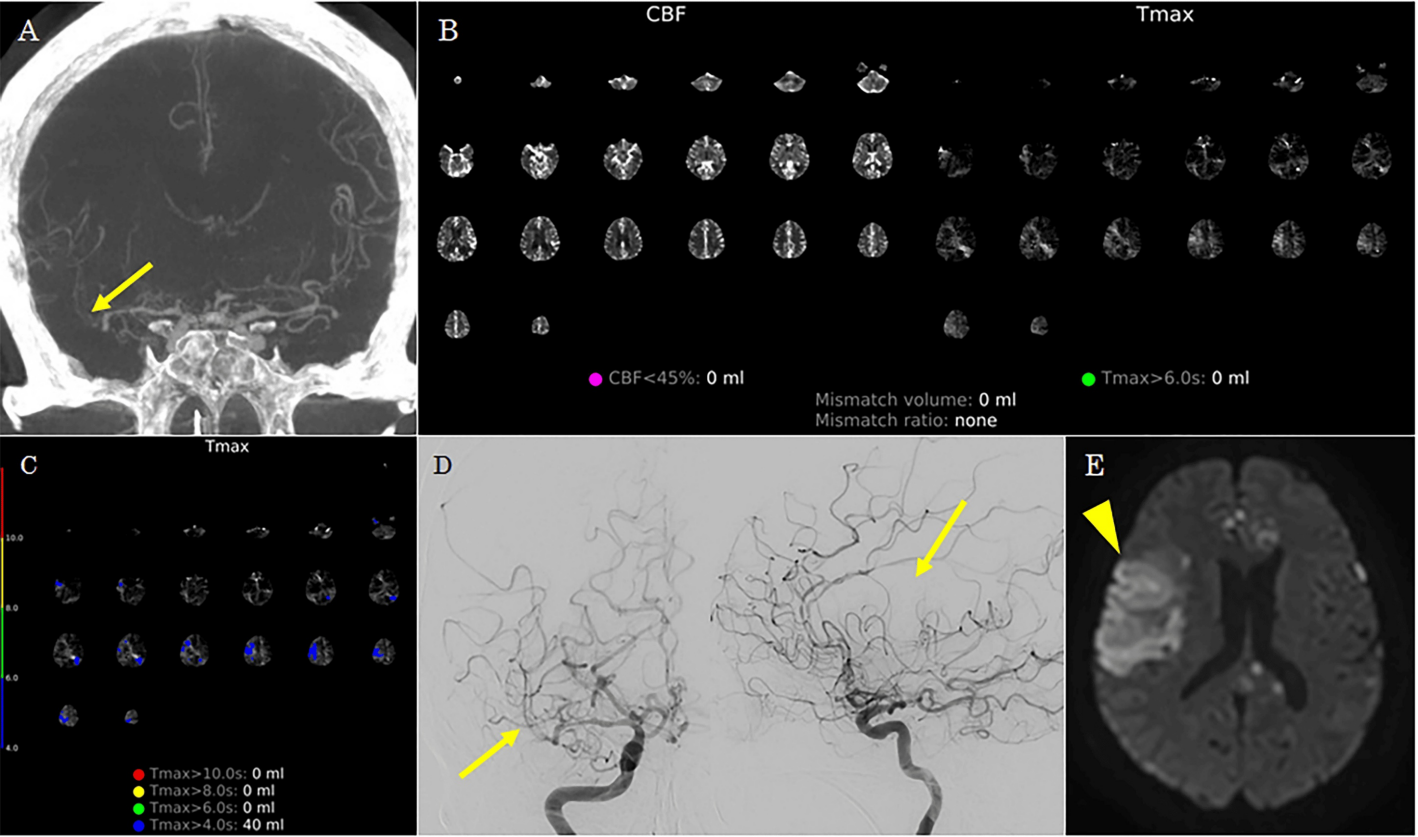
Case 2. (A) CT angiography-like images from RAPID ANGIO showing diffuse narrowing of the right middle cerebral artery (arrow). (B) Automated perfusion maps from RAPID ANGIO show no significant infarct core (cerebral blood flow <45%) or hypoperfused tissue with Tmax >6 seconds. (C) Detailed Tmax maps from the RAPID ANGIO suggest mildly delayed perfusion (Tmax >4 seconds) in the right middle cerebral artery. (D) Right carotid angiography demonstrating diffuse vasospasm of the right middle cerebral artery (arrow), which did not improve following intra-arterial administration of fasudil hydrochloride. (E) Post-procedural diffusion-weighted MRI revealed an infarction in the region of mild hypoperfusion previously suspected in RAPID ANGIO.
